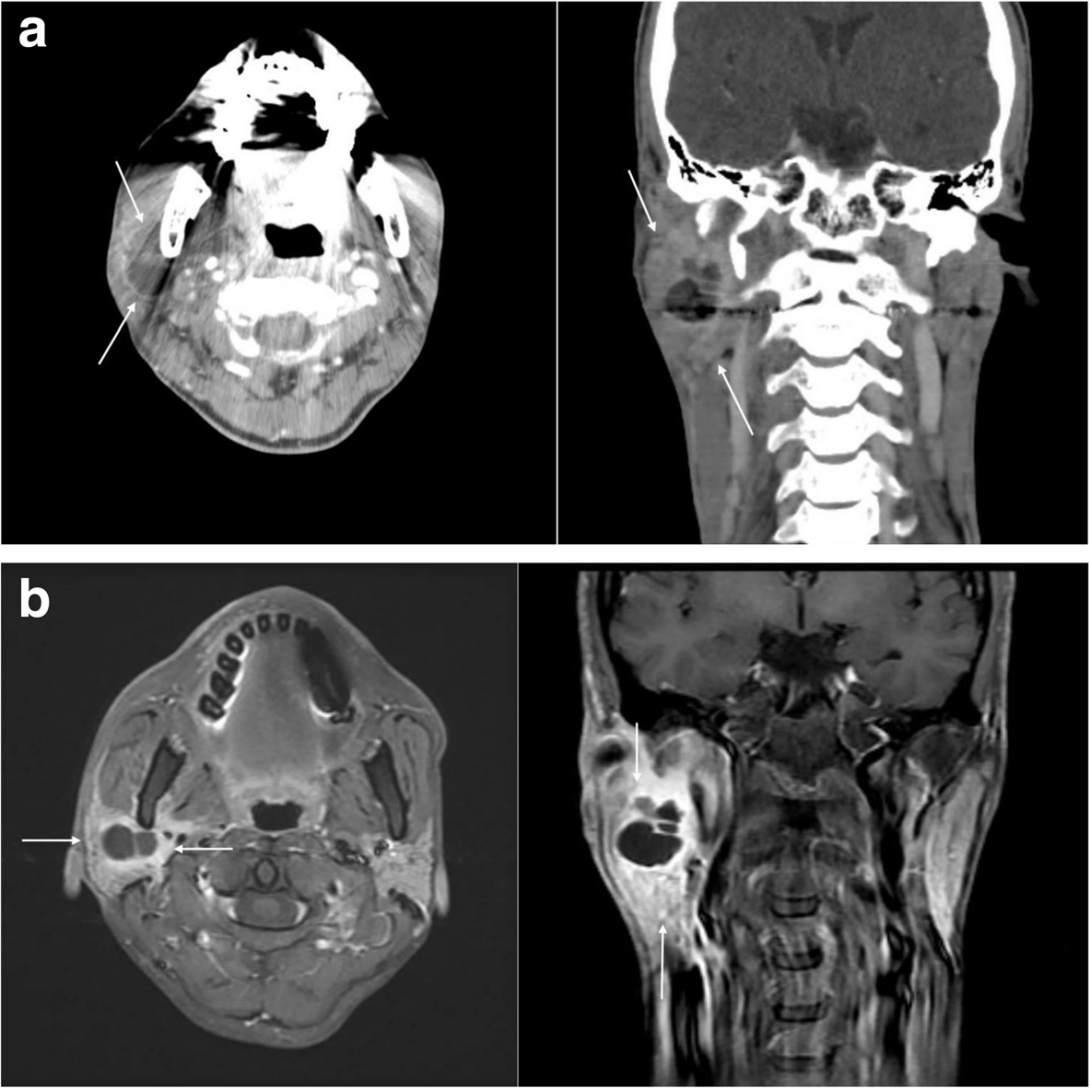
Pre-operative radiographic images of the case 1 patient. Parotid CT (a) and MRI (b) showed glandular enhancement with lobulating cystic lesions in the right parotid gland
